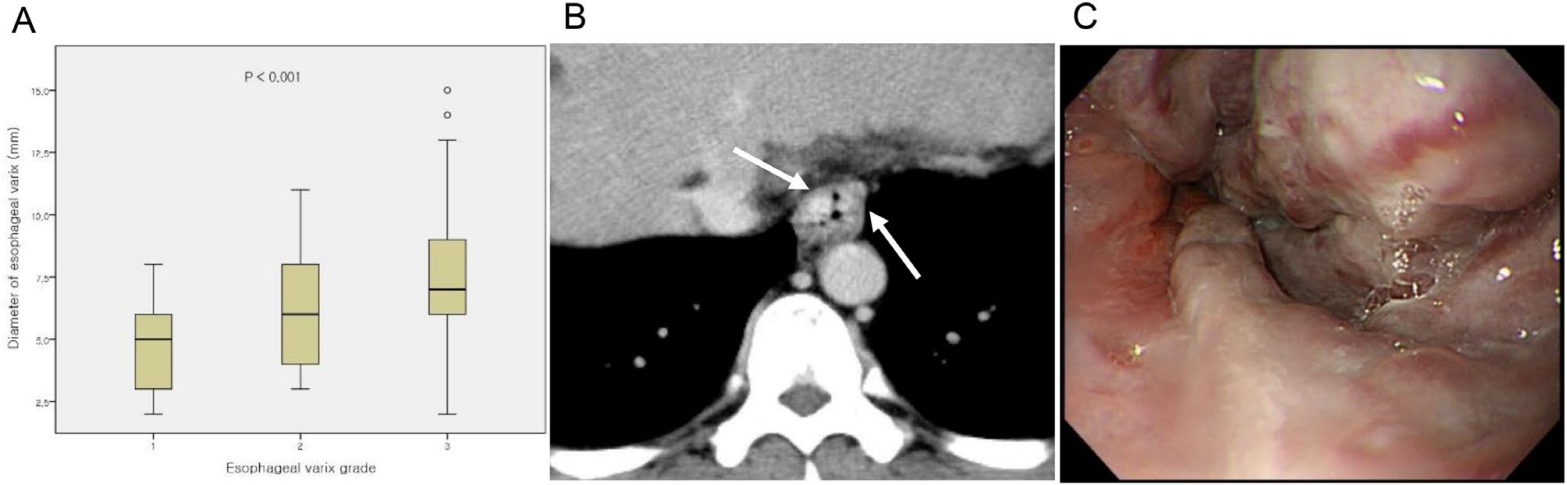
A Relationship between CT variceal diameter and endoscopic grading. B, C 44-year-old man with liver cirrhosis and large esophageal varices. Portal phase CT image of esophagus shows multiple nodular, enhancing, intraluminally protruding lesions (arrows) within esophagus wall. In this patient, largest varix was measured as 10.7 mm. Endoscopic image shows multiple large varices
